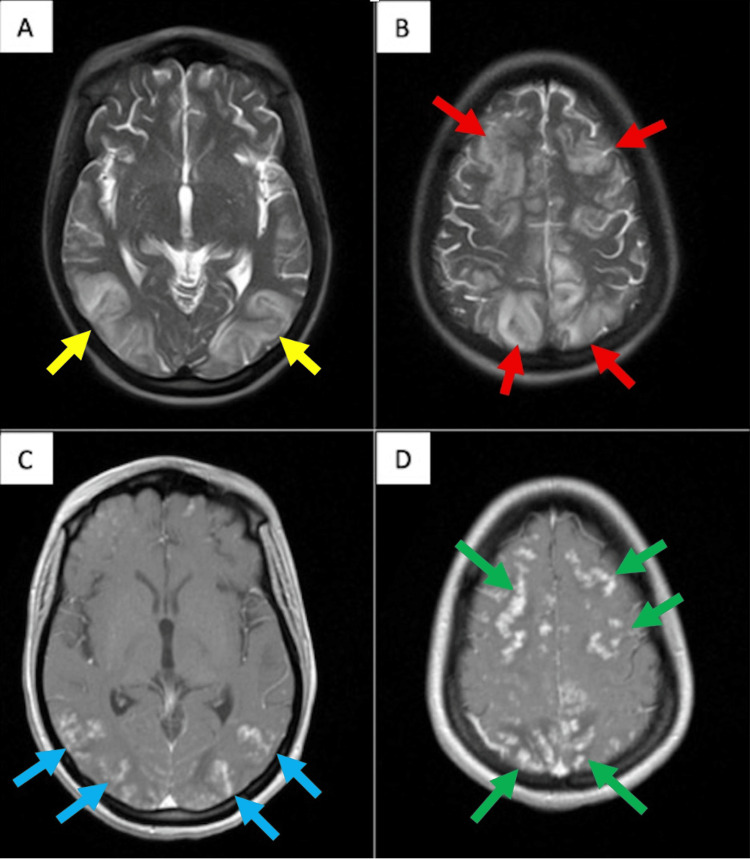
Brain MRI showing features atypical for posterior reversible encephalopathy syndrome (PRES) (A) Axial Half-Fourier Acquisition Single-Shot Turbo Spin-Echo (T2/HASTE) image showing bilateral parieto-occipital hyperintensities (yellow arrows); (B) Axial T2/HASTE image showing bilateral frontal and parietal hyperintensities (red arrows); (C) Post-contrast axial T1 image demonstrating nodular enhancement in the bilateral parieto-occipital lobes (blue arrows); (D) Post-contrast axial T1 image demonstrating nodular enhancement in the bilateral frontal and parietal lobes (green arrows).
